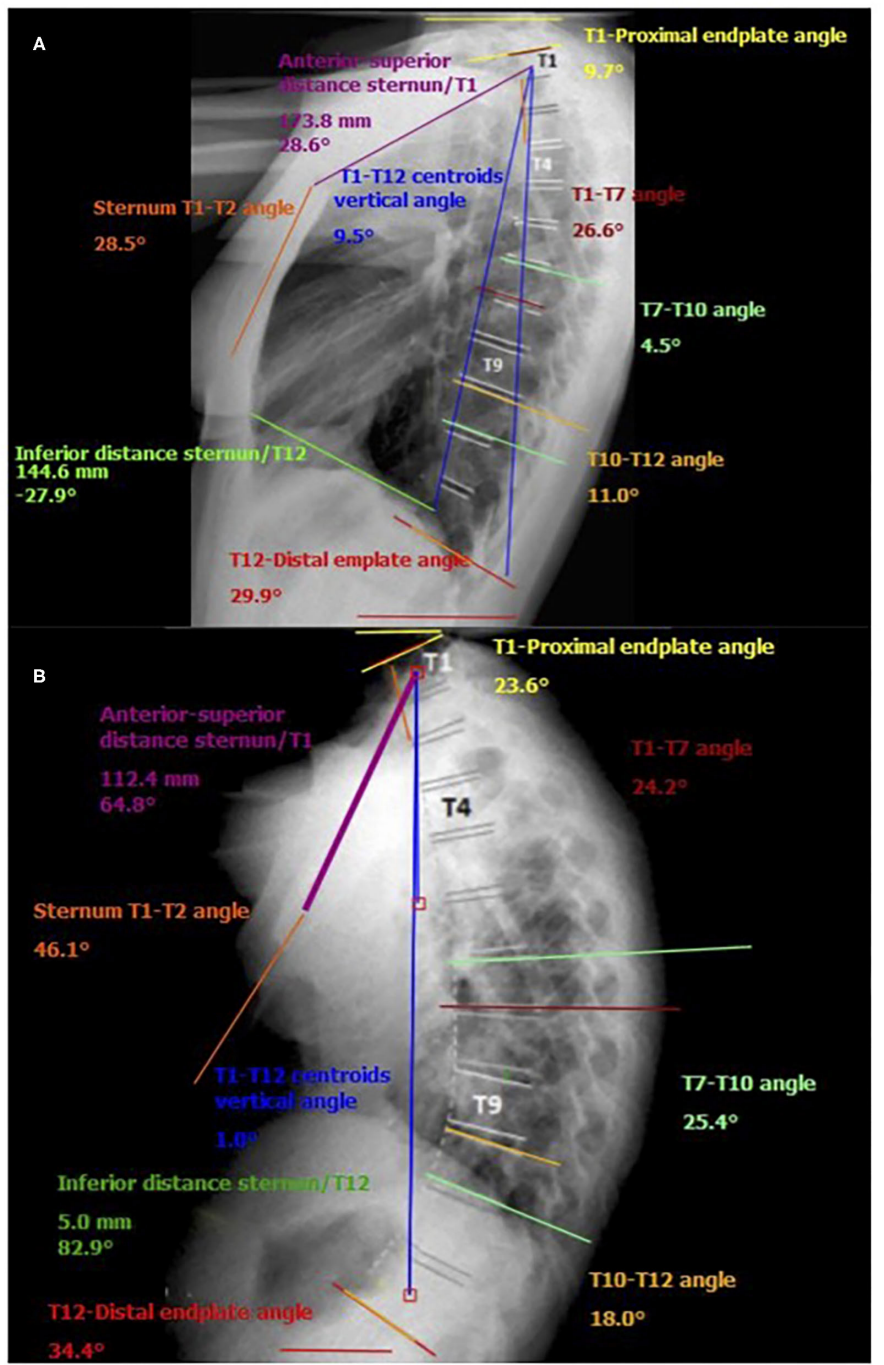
Example of measurements taken by the surgimap software from the lateral radiographs of the thoracic spine in one of the participants. (A) forced inspiration; (B) forced exhalation.
